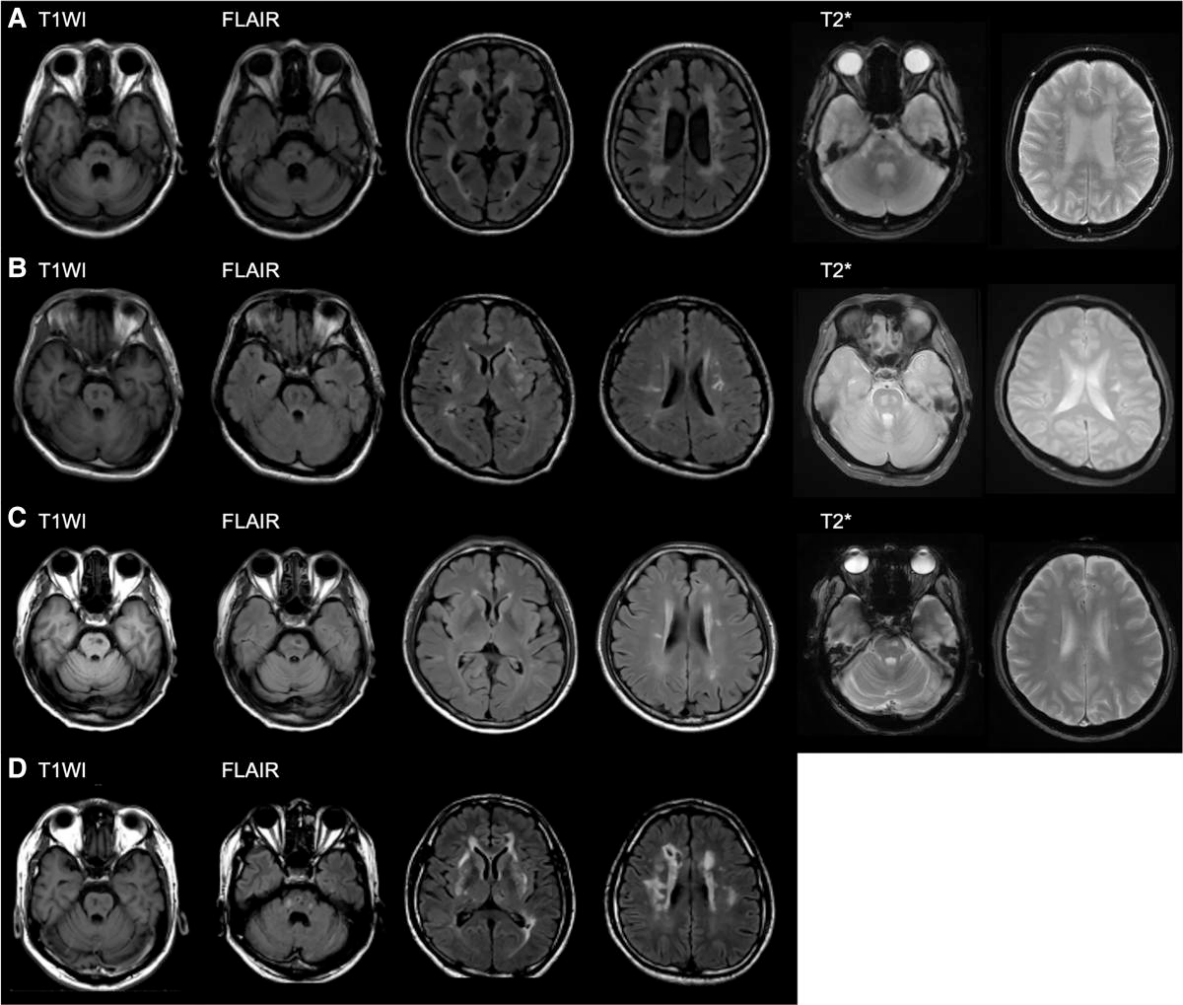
MRI features of the probands in Family 1–3. (A–D) MRI scan was performed using the genetically proven probands at 1.5 T in Family 1 and at 3 T in Family 2 and 3. The examination was performed 9 years after the onset for F1-IV-2 (A), 7 years after the onset for F1-IV-6 (B), 8 years after the onset for F2-II-3 (C) and 12 years after the onset for F3-II-2 (D). T1-weighted images (T1WI) show low intensity areas located in the pons and white matter in all four cases. The pontine atrophy was observed in the autopsy case (F1-IV-2). Multiple infarctions were observed in the pons on fluid attenuated inversion recovery and corresponded to the low intensity areas depicted on T1WI. Every patient showed at least one oval shape lesion in the pons. Bilateral white matter hyperintensity was also observed in all cases.
